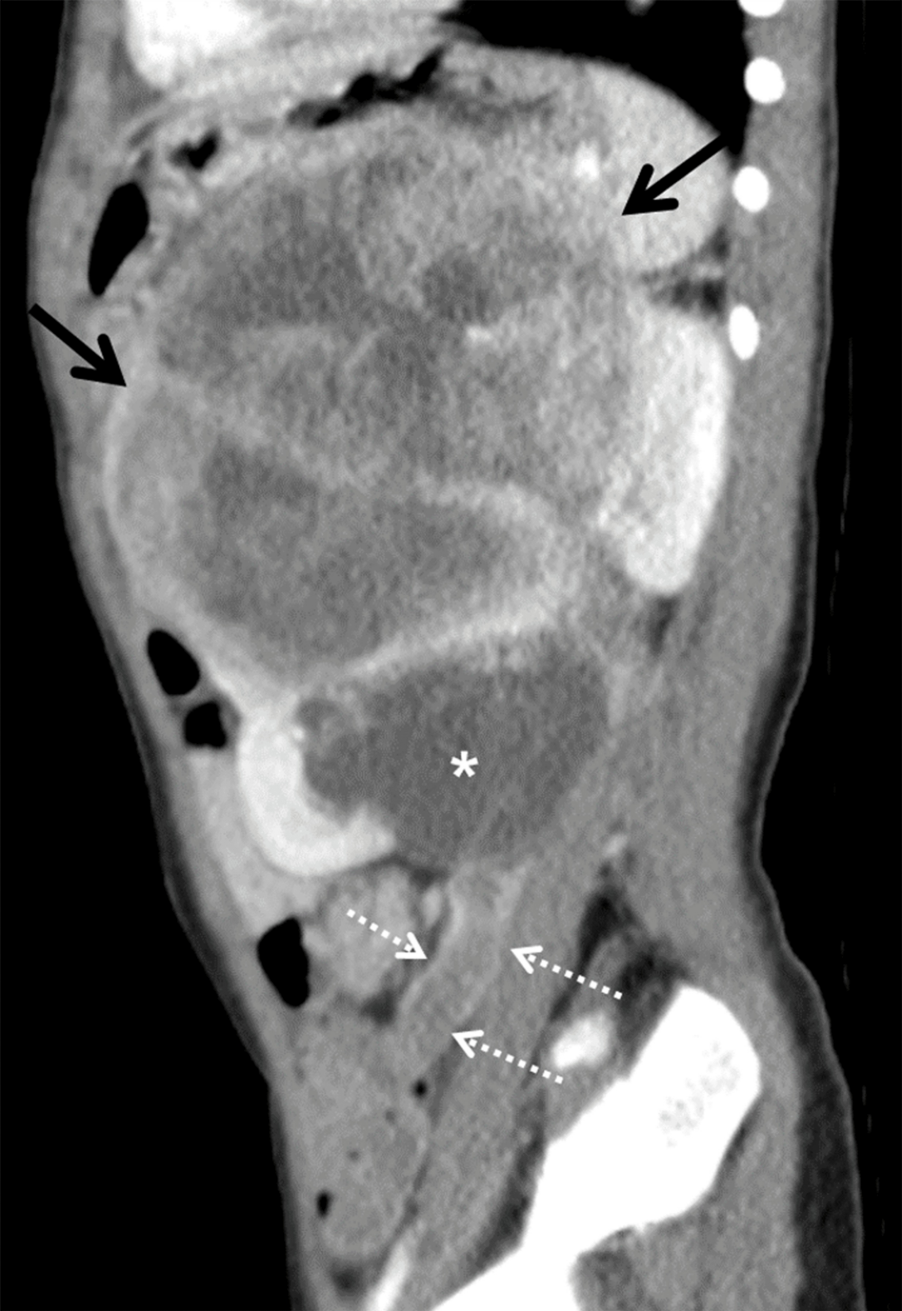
The sagittal CT image shows WT in the left kidney (arrows) with a tumor extension into the dilated left renal pelvis (asterisk) and the left ureter (dashed white arrows). CT: computed tomography; WT: Wilms tumor
